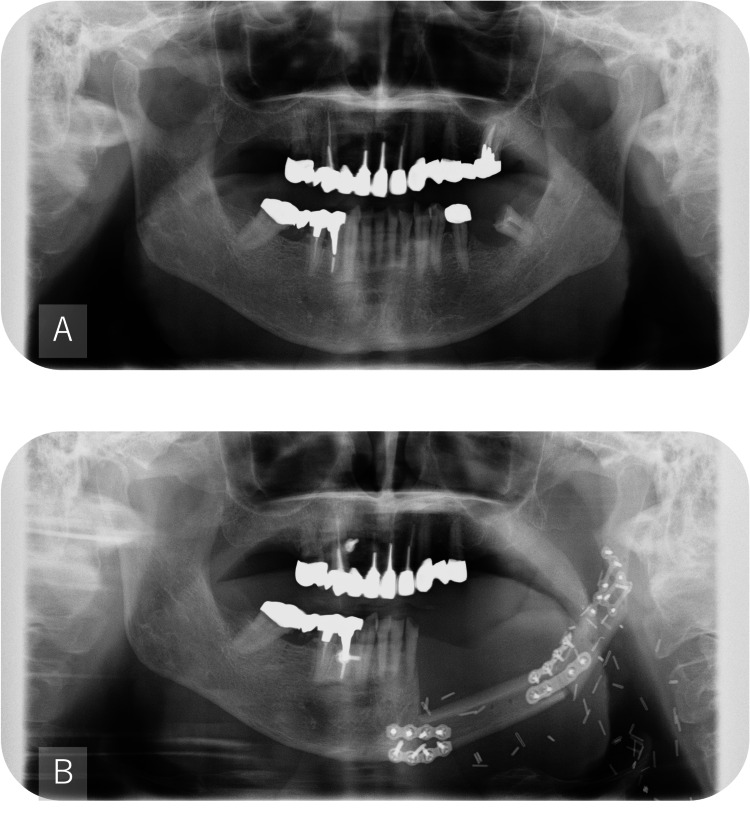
Panoramic radiographs A: Preoperative; B: One month postoperative
